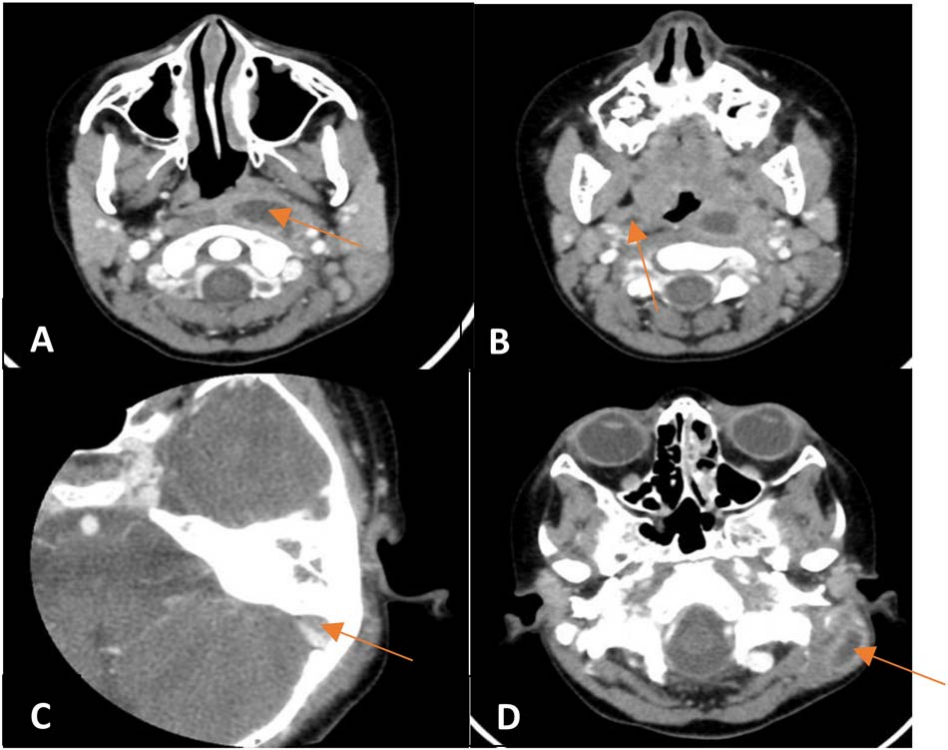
Enhanced CT scan of the temporal bones and neck showing (A) a left parapharyngeal space fluid collection with enhancing walls, (B) a small right parapharyngeal collection with minimally enhancing walls, (C) an intracranial extension of mildly enhancing lesion to subdural space and (D) a left mastoid bone showing lateral wall cortical disruption with adjacent retro-auricular irregular collection with enhancing walls.
